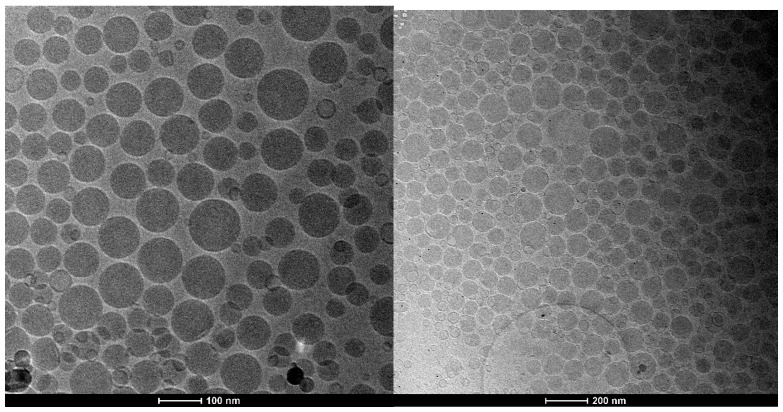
Cryo-TEM projection images of dryTom-Ne (left) and dryTom-Ne-CT (right).
